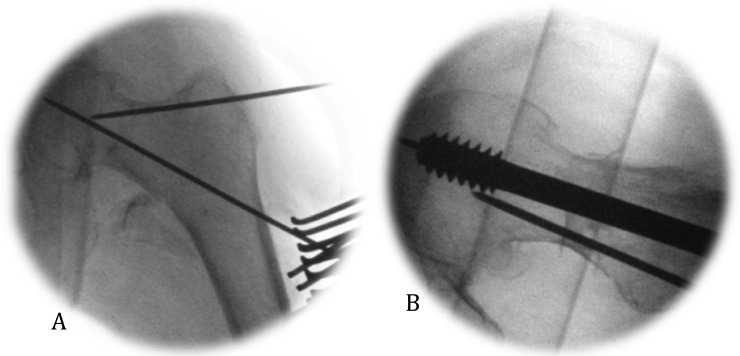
(A) Anti-rotation wire used at forty five degrees to the guide wire to facilitate stability during reaming and tapping of femoral neck.(B) Lateral radiograph of anti-rotation wire preventing rotation and fracture displacement during insertion of screw.
